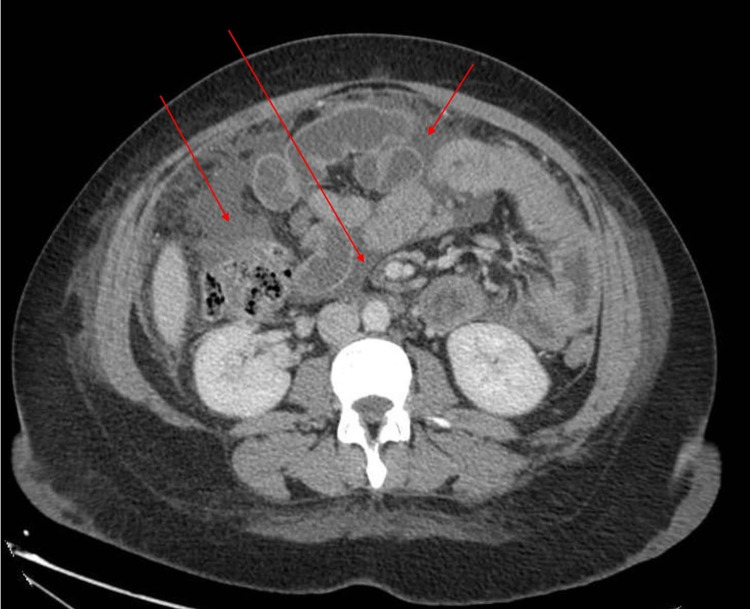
Axial CT abdomen demonstrating multiple intra-abdominal collections among bowel loops (red arrows)
